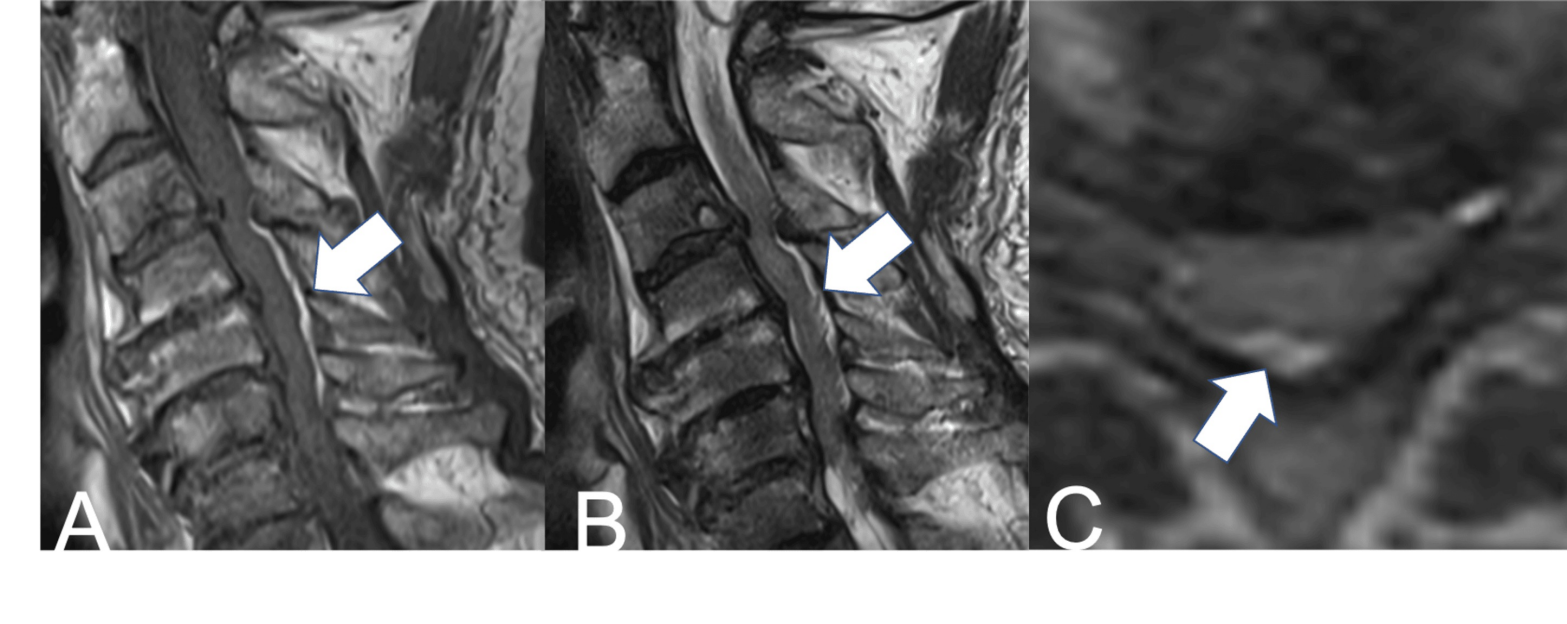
(A) Sagittal T1-weighted MRI at the time of deterioration; (B) Sagittal T2-weighted MRI at the time of deterioration; (C) Axial T2-weighted MRI at the time of deterioration Arrows show high signal intensity in the epidural area on both T1-weighted and T2-weighted images.
